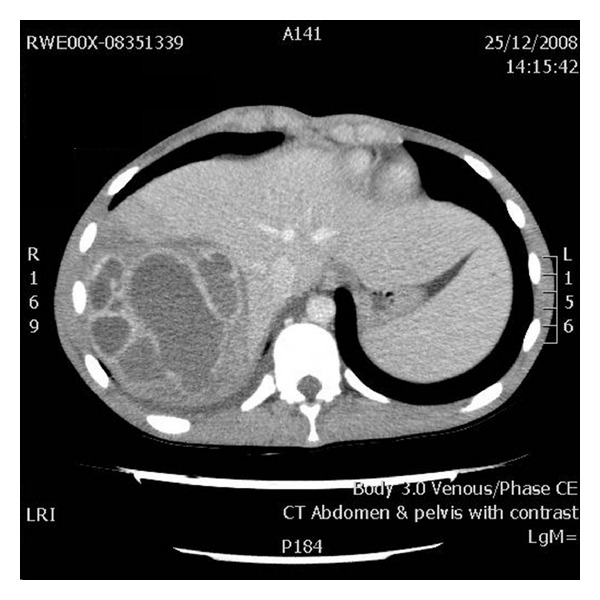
CT scan demonstrating multiloculated liver abscess containing at least 10 locules.
